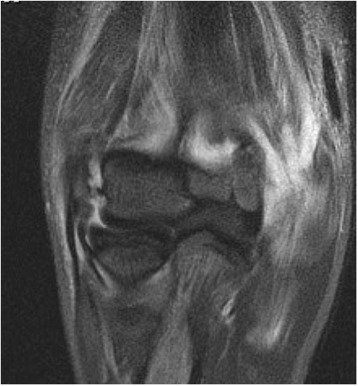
Coronal view in T2-weighted MRI after simple elbow dislocation: The LCL was rated to be partially torn (2×) and completely torn (2×)
